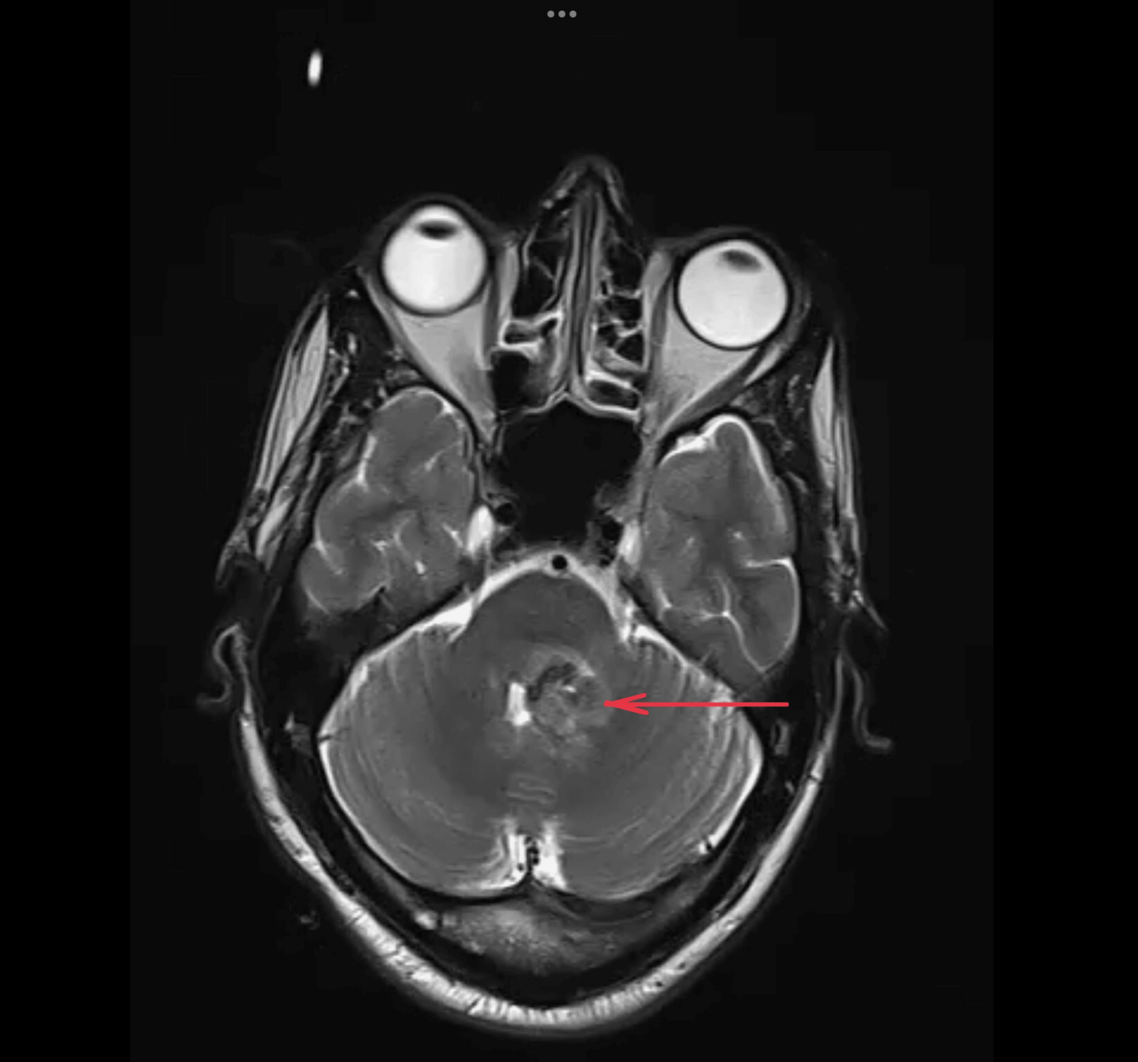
MRI brain: T2-weighted image showing heterointense area involving the left posterior aspect of the hemipons extending to left middle cerebellar peduncle and into the adjacent cerebellum, causing mass effect in the form of mild displacement and compression of fourth ventricle to the right pointed using red arrows MRI: Magnetic resonance imaging
